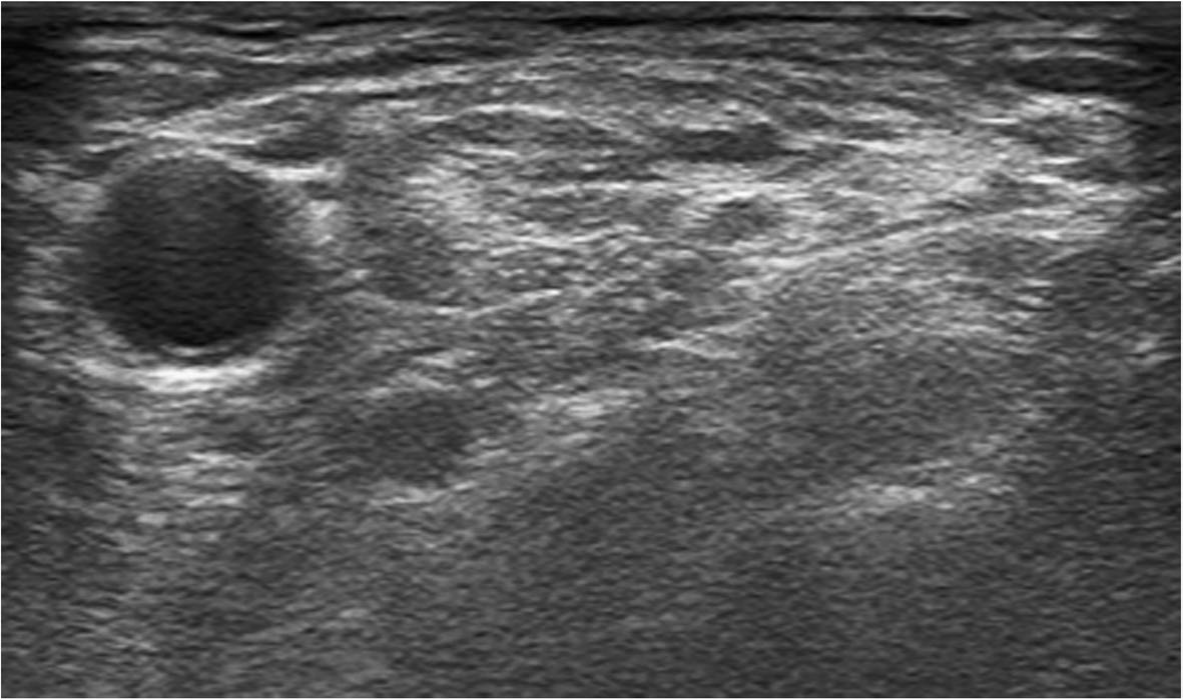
Ultrasound image of interscalene brachial plexus block. ICA, internal carotid artery; ASM, anterior scalene muscle; MSM, middle scalene muscle; BP, brachial plexus; N, needle
